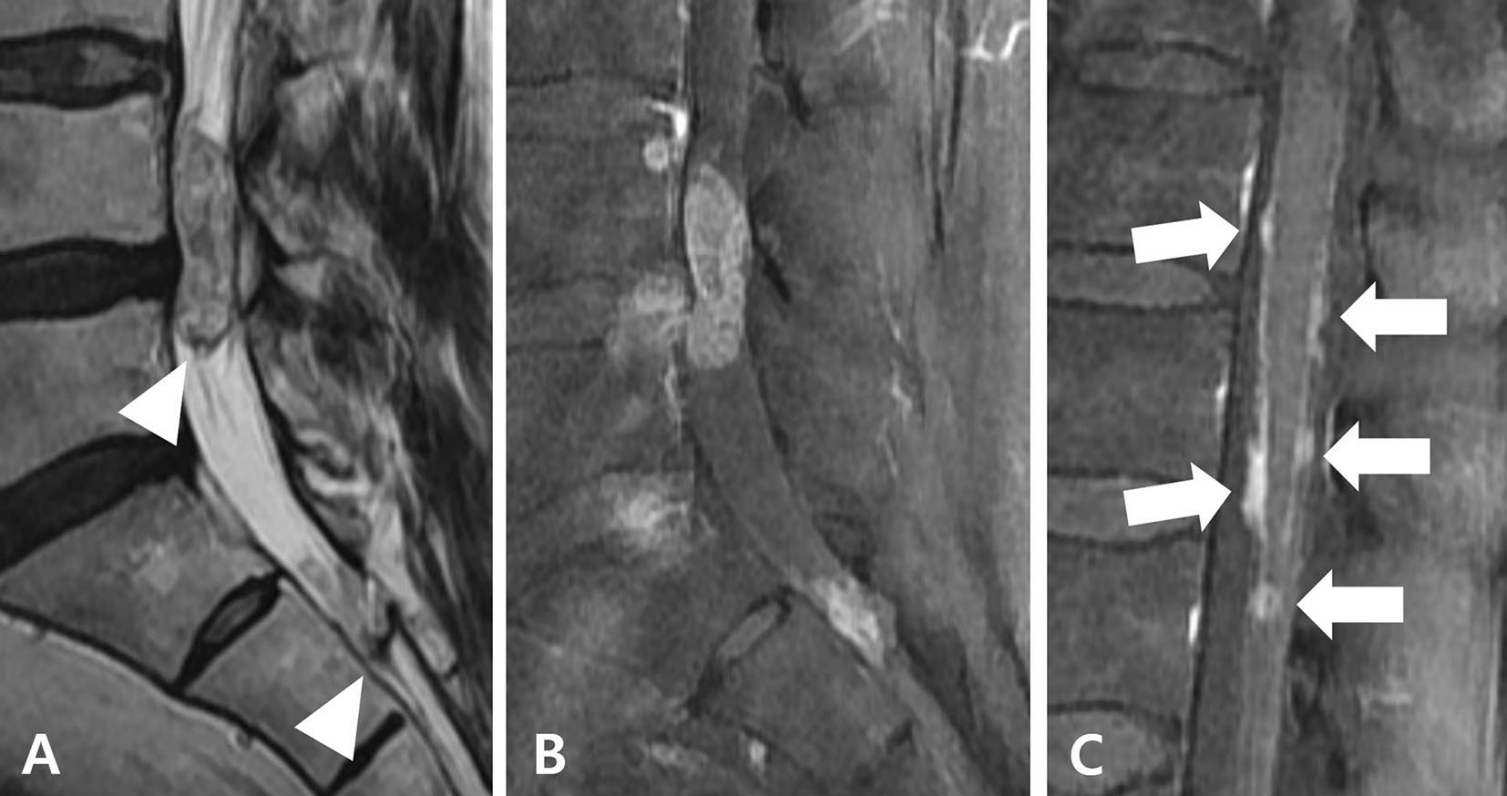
A myxopapillary ependymoma in a 65-year-old male patient at the L4-5 level. Sagittal T2-weighted (A) and fat-suppressed contrast-enhanced T1-weighted (B) images show a 4.2 cm-sized intradural mass at the L4-5 level with a leptomenigeal seeding at the distal dural sac. Both lesions demonstrate intratumoral T2 dark signal (arrowheads) and diffuse heterogeneous enhancement pattern. Sagittal fat-suppressed contrast-enhanced T1-weighted image at the thoracolumbar junction level (c) also shows multiple leptomeningeal seedings along the spinal cord surface.
